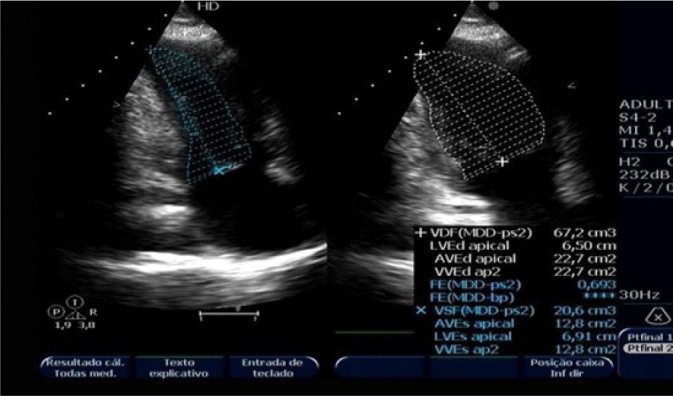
Echocardiogram (diastole and systole) from April 3, 2015 showing left ventricular abnormality, with the typical Takotsubo format.
